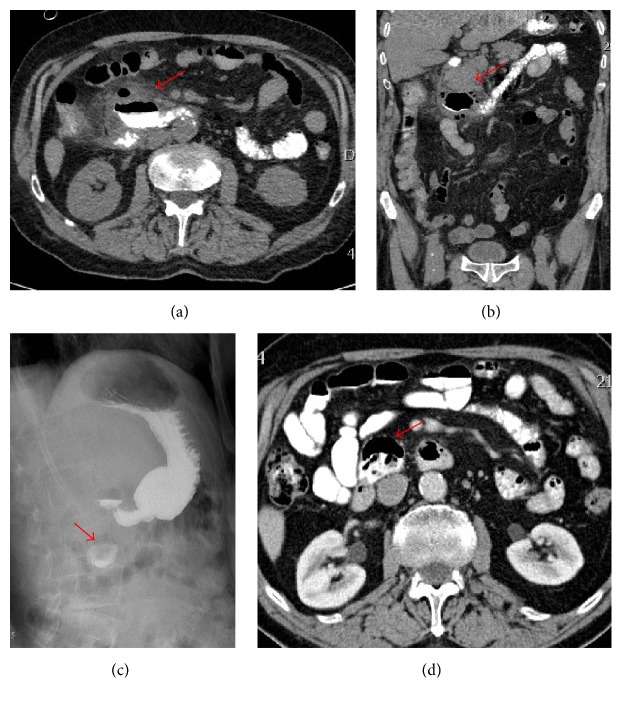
(a & b) Computed Tomography of the abdomen, with PO contrast, showing the evidence of a duodenal diverticulum with contrast extravasation, surrounded by fat stranding and air pockets (arrow), suggestive of perforation ((a) axial; (b) coronal). (c) Gastrografin swallow fluoroscopy, upon follow-up, showing the presence of contrast within the diverticulum (arrow) and absence of any extravasation. (d) Enhanced CT scan with IV and PO contrast showing the diverticulum with absence of extravasation and minimal air pocket with fat stranding (arrow).
